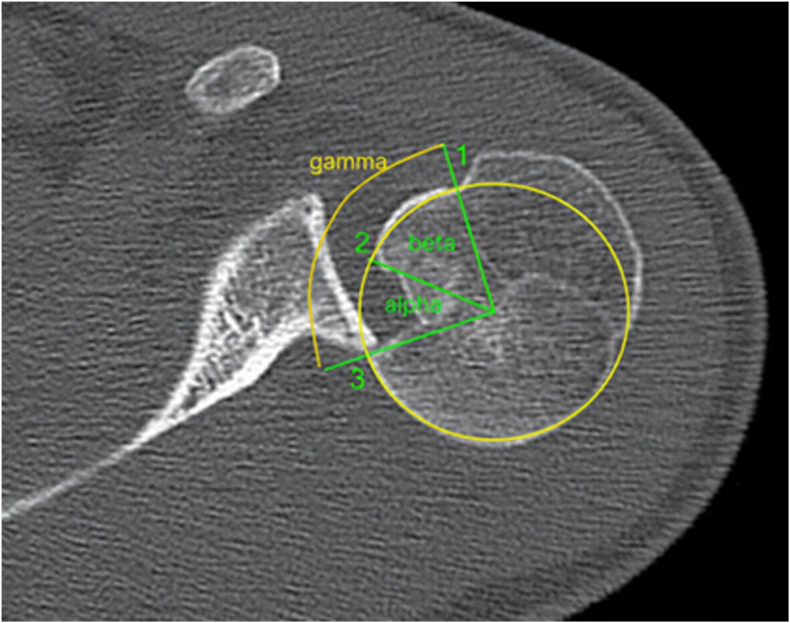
CT scan of the left shoulder joint with locked posterior dislocation. The alpha angle is measured between the anterior (2) and posterior (3) margins of the RHSD and characterizes the defect size. The beta angle is measured between the intertubercular sulcus (1) and the anterior margin (2) of the defect. The gamma angle is measured between the intertubercular sulcus (1) and the posterior margin (3) of the RHSD and represents the sum of alpha and beta angles. RHSD: reverse Hill-Sachs defect.
